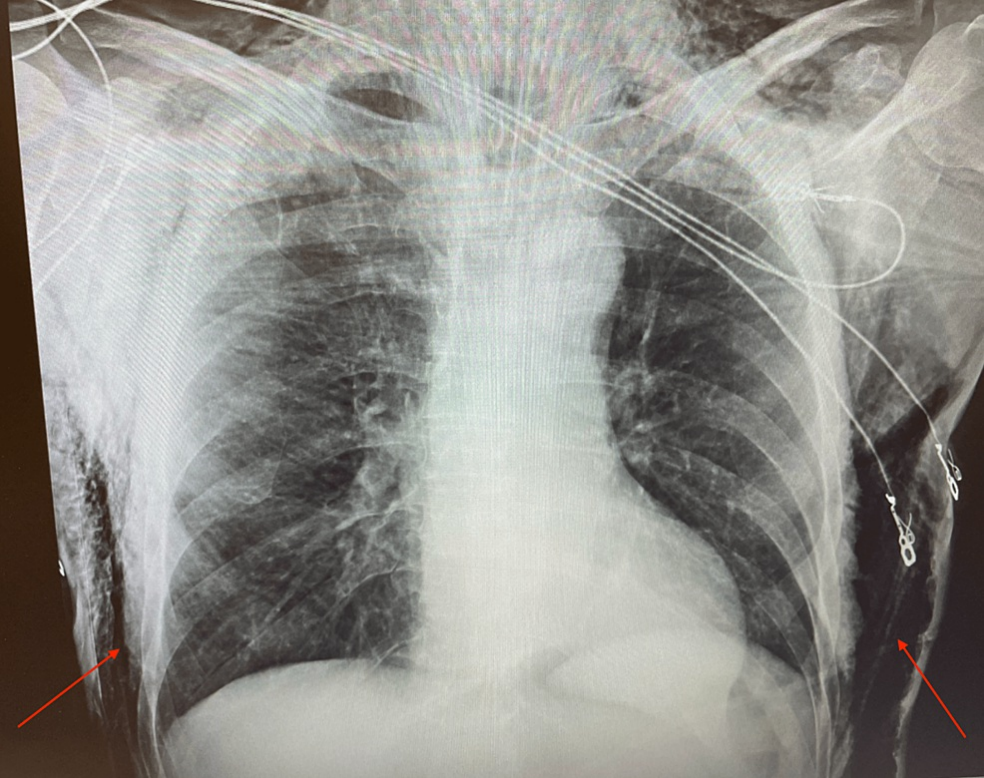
Chest X-ray obtained postoperatively in the ICU, which demonstrated extensive subcutaneous emphysema in the exterior borders of the thoracic walls.
